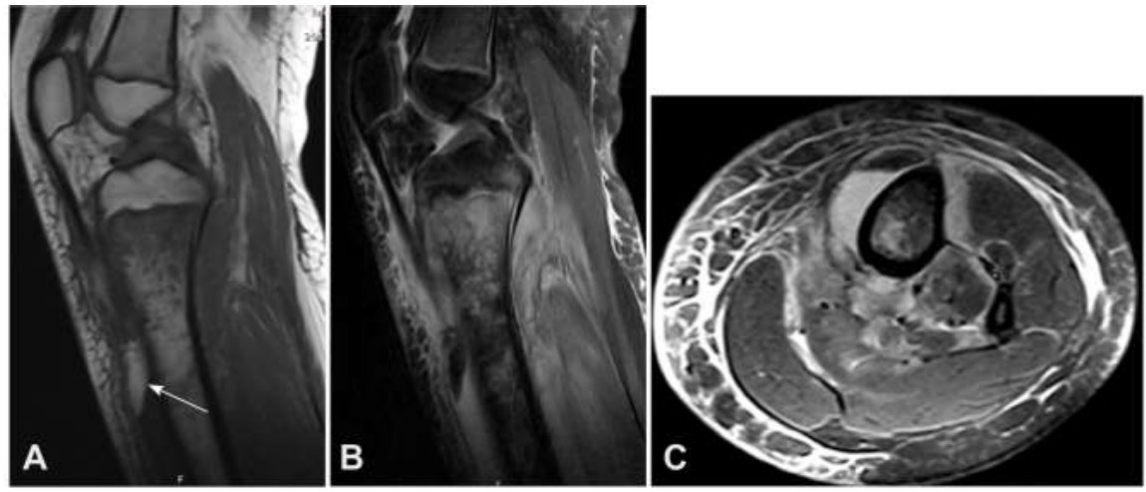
Magnetic resonance imaging (MRI) of the fluid level. (A & B). MRI sagittal T1WI (A) and fat suppression (FS) T2WI (B) demonstrated the lipid level (arrow) in the front of the upper segment of the tibia, with T1WI showing an equal signal between the lower part of the fluid level and the muscle, and a high signal similar to fat in the upper part. C. MRI plain scan cross-sectional short-term reversal recovery sequence (STIR) T2WI showed a high signal similar to liquid in the lower part and a low signal in the upper part.
